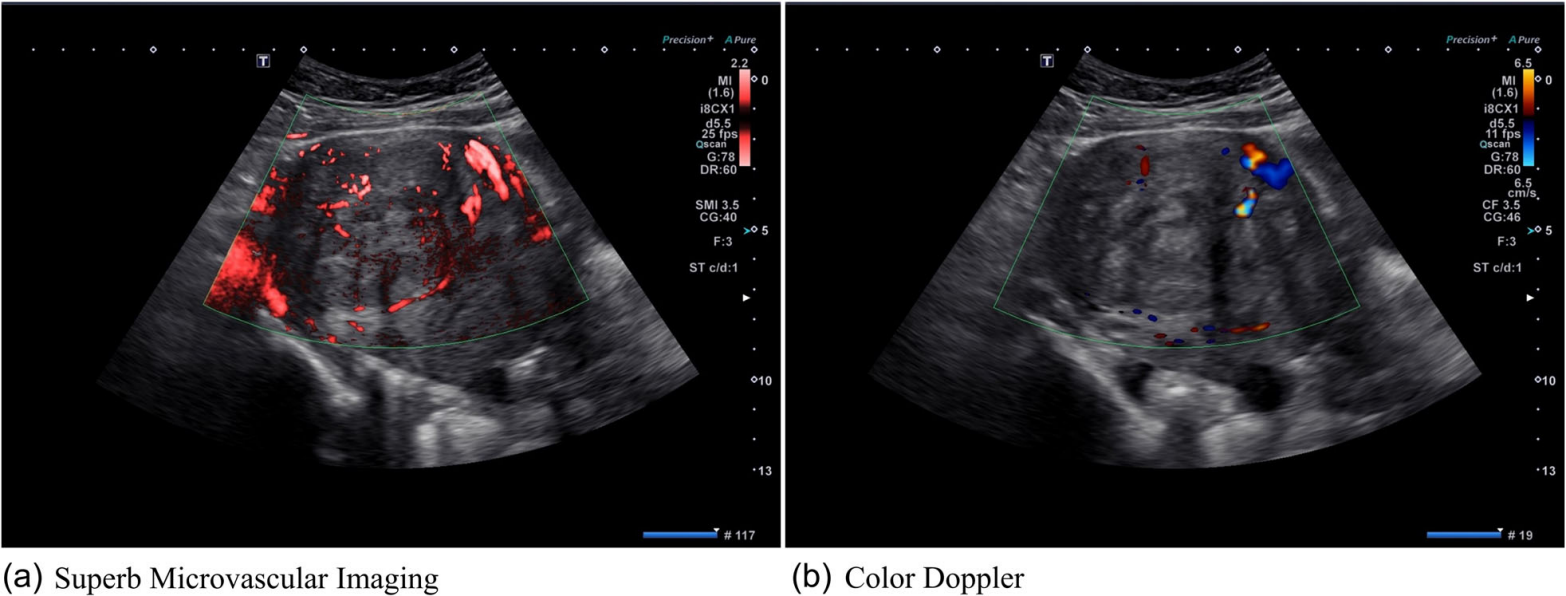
Superb microvascular imaging and color Doppler imaging. (a) Superb microvascular imaging demonstrated strong blood flow in the presumed vascular capsule surrounding the fibroid, as well as weak blood flow within the fibroid. (b) Color Doppler revealed a large blood vessel in the upper‐right corner of the fibroid; however, other small blood vessels were barely visible.
